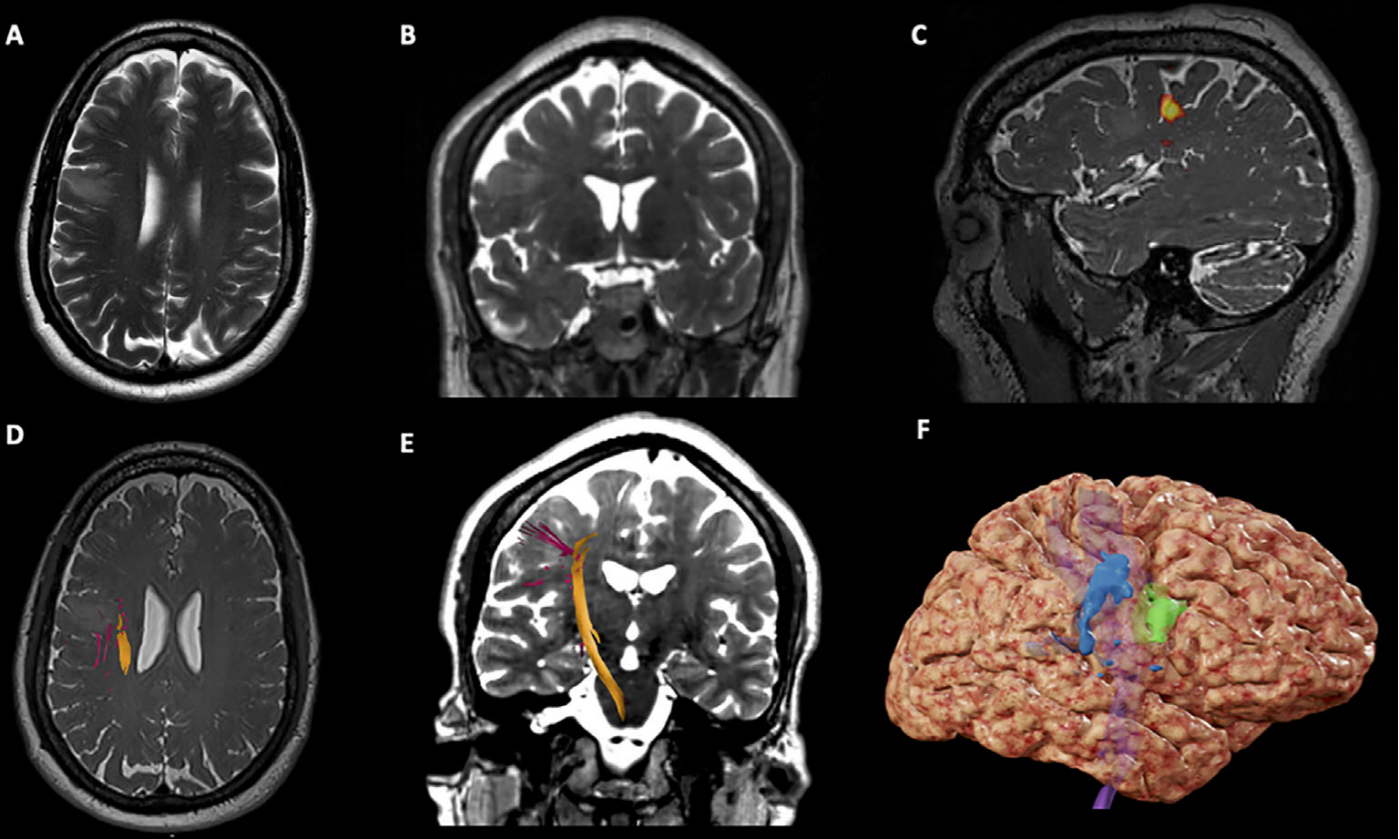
Case 1 Surgical Plan. (A) Axial T2-weighted imaging showing an hypointensity in the right middle frontal gyrus, anterior to the precentral gyrus; (B) Coronal T2-weighted imaging showing an hypointensity in the right middle frontal gyrus; (C) Sagittal T2*-weighted imaging showing BOLD activation for lip purse test (yellow) on the lateral aspect of the right precentral gyrus; (D) Axial T2*-weighted imaging showing the right Corticospinal Tract (CST) on the deep and posterior margins of the tumor; (E) Coronal T2-weighted imaging showing the right CST descending from the precentral gyrus in the posterior aspect of the tumor; (F) 3D brain reconstruction showing the BOLD activation for lip purse segmented (in blue), the segmented tumor (green) and the CST deep (in purple).
